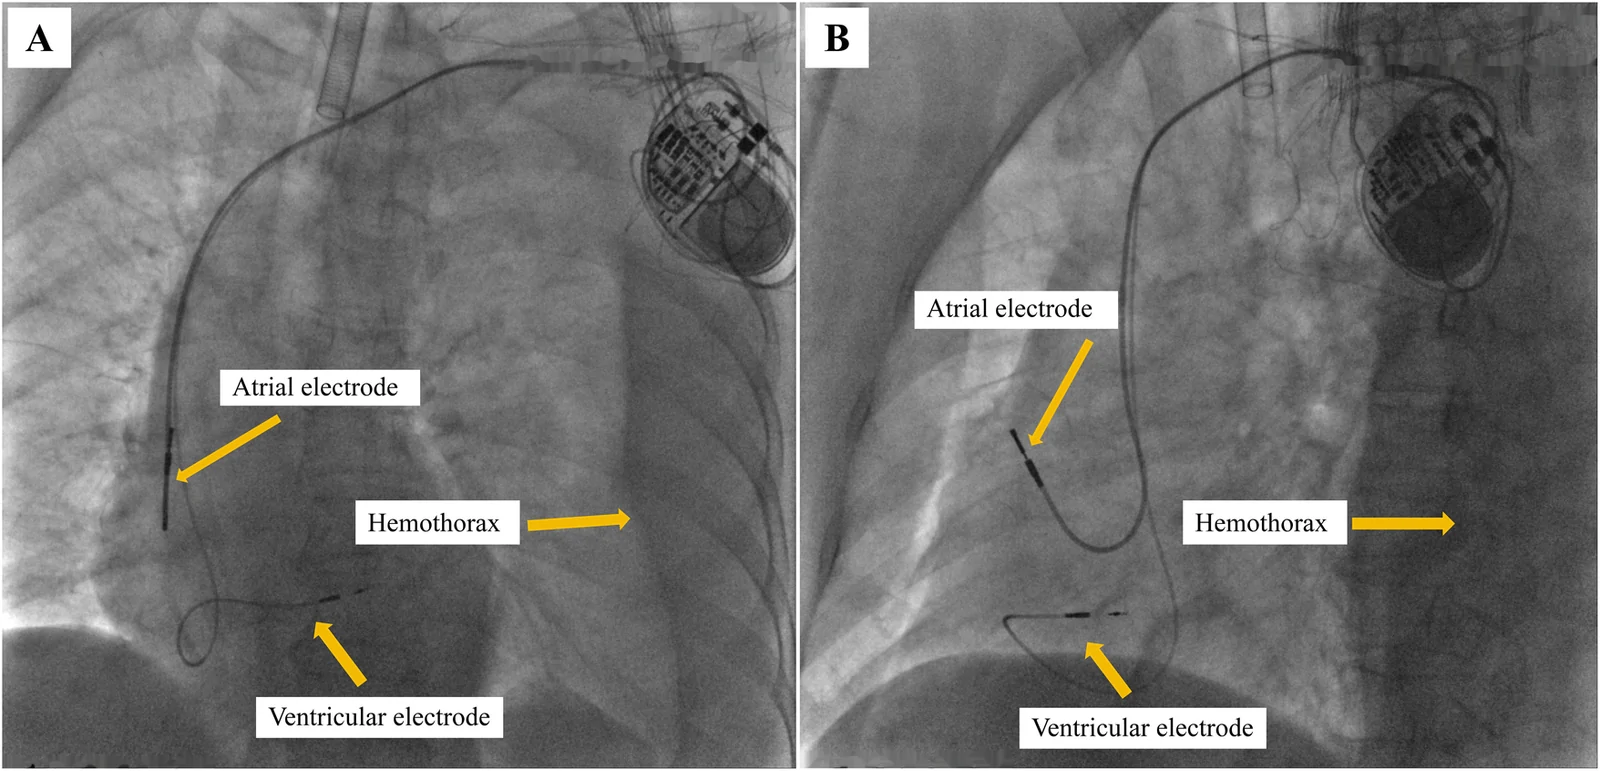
Anteroposterior (A) and 45° left anterior oblique (B) intraprocedural fluoroscopic radiographs demonstrating diffuse homogeneous opacification of the entire left hemithorax, consistent with severe postprocedural hemothorax. The modified radiograph excludes overlay cardiac device graphics to provide unobscured visualization of the left clavicular region, subclavian venous puncture site, proximal lead trajectory, and osseous landmarks. The labeled yellow arrows denote the atrial lead and ventricular lead, respectively.
